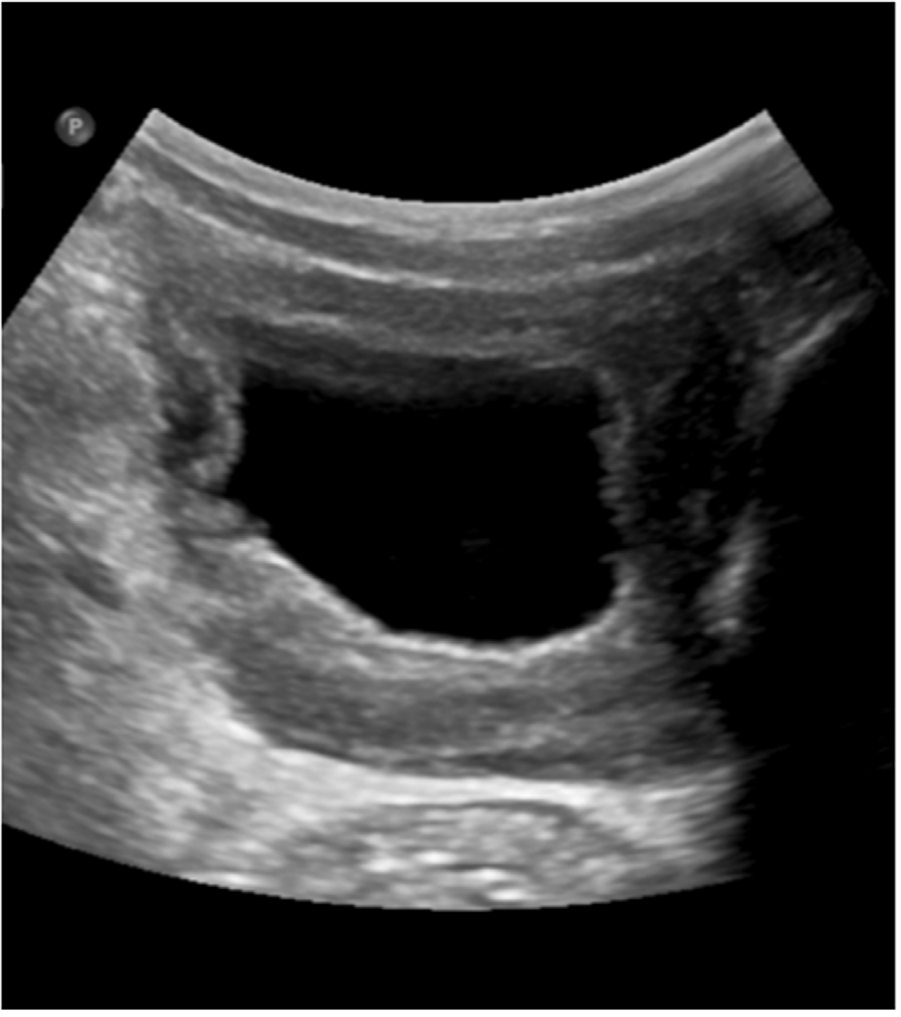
Follow up ultrasound of the bladder showing complete resolution of the pelvic mass.
